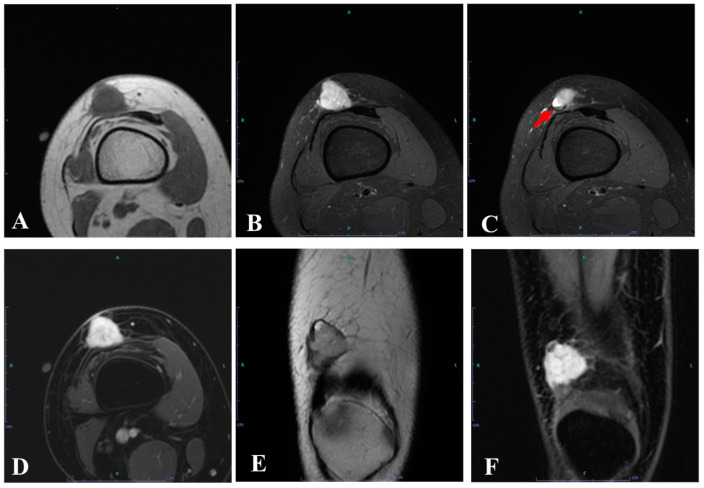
Over 8 years, the mass gradually increased in size, and the patient experienced occasional pain, prompting a return to the local clinic. Aspiration of the mass yielded no contents, suggesting that it was a solid lesion. The patient was then referred to our hospital for further evaluation. MRI at our hospital showed a tumor located subcutaneously adjacent to the vastus lateralis, with iso-intensity signals on T1WI compared with muscle (A) and heterogeneous high-intensity signals on T2WI (E) and fat-suppressed T2WI (B,C). The tumor was multiloculated, with a pseudocapsule (E), and contained a fluid level (C; red arrow) and peritumoral edema (B). It measured 22 × 18 × 30 mm. A heterogeneous enhancement effect was observed, excluding the cystic structure (D,F). An ultrasound-guided needle biopsy suggested angiomatoid fibrous histiocytoma (AFH). No metastasis was confirmed on contrast-enhanced CT. Characteristic MRI findings in AFH include a pseudocapsule, multilocular area, cystic area, fluid levels, and peritumoral edema [1,2]. However, since these findings can also be seen in various other soft tissue tumors, their specificity is low. Additionally, the sensitivity of these findings has not been evaluated in many cases, and cases without these findings have been reported [3], warranting further investigation. Contrast enhancement is generally seen in all cases, but enhancement patterns vary [1,2]. Considering that the average size of AFH tumors is generally small, there is a high possibility of overlooking the characteristic features, even with MRI. In our case, the characteristic findings were relatively easily identified on MRI at our hospital; however, even when analyzing the MRI from 8 years prior retrospectively after the diagnosis of AFH, several AFH features could be identified. It is extremely difficult to identify these features at a stage when the diagnosis has not yet been made. Differential diagnoses based on MRI include benign tumors (such as hemangioma and hematoma) and malignant tumors (such as synovial sarcoma and myxofibrosarcoma), making accurate diagnosis through imaging alone difficult [1]. Cystic tumors can occur in various locations, including the knee, and ganglions, bursitis, and atheroma are commonly encountered in daily practice. When determining whether a tumor is cystic, greater caution should be exercised when relying solely on plain MRI. In cystic tumors such as ganglions, there is no internal blood flow, giving contrast-enhanced MRI a significant advantage in differentiation. When there is an increase in tumor size or appearance of symptoms, contrast-enhanced MRI or at least Doppler ultrasound for blood flow evaluation should be performed.
